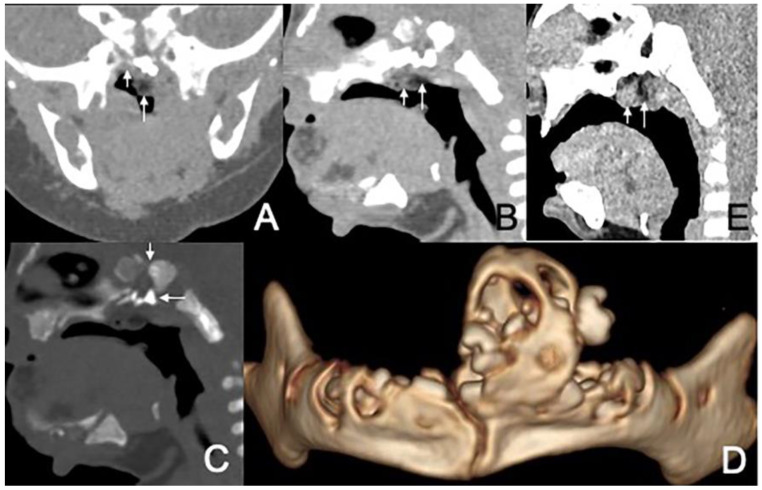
CT of a 1-month and 6-day girl presenting shortly after birth due to being unable to suck and having a mandibular mass. (A,B) Coronal and sagittal CT, displayed in the soft-tissue window, show the irregular fat (short arrow) and soft tissue (long arrow) density mass originating from the back side of the left soft palate. (C) Sagittal CT, displayed in the bone window, shows that there were multiple irregular dense shadows (long arrow) above the mass that were not seen, were excised during the transnasopharyngeal plasma surgery, and were probably ectopic teeth. In addition, the central bone defect of the sphenoid body was considered the remnant of craniopharyngeal canal development (short arrow). Intraoperatively, the nasopharyngeal apex wall was found intact, and no clear fluid outflow was observed. This patient was associated with cleft palate (not shown). (D) 3D reconstruction CT showing the associated ameloblastic fibro-odontoma of the mandible. (E) Sagittal CT after 3 years, displayed in a soft window, shows that the volume of the mass was increased slightly.
